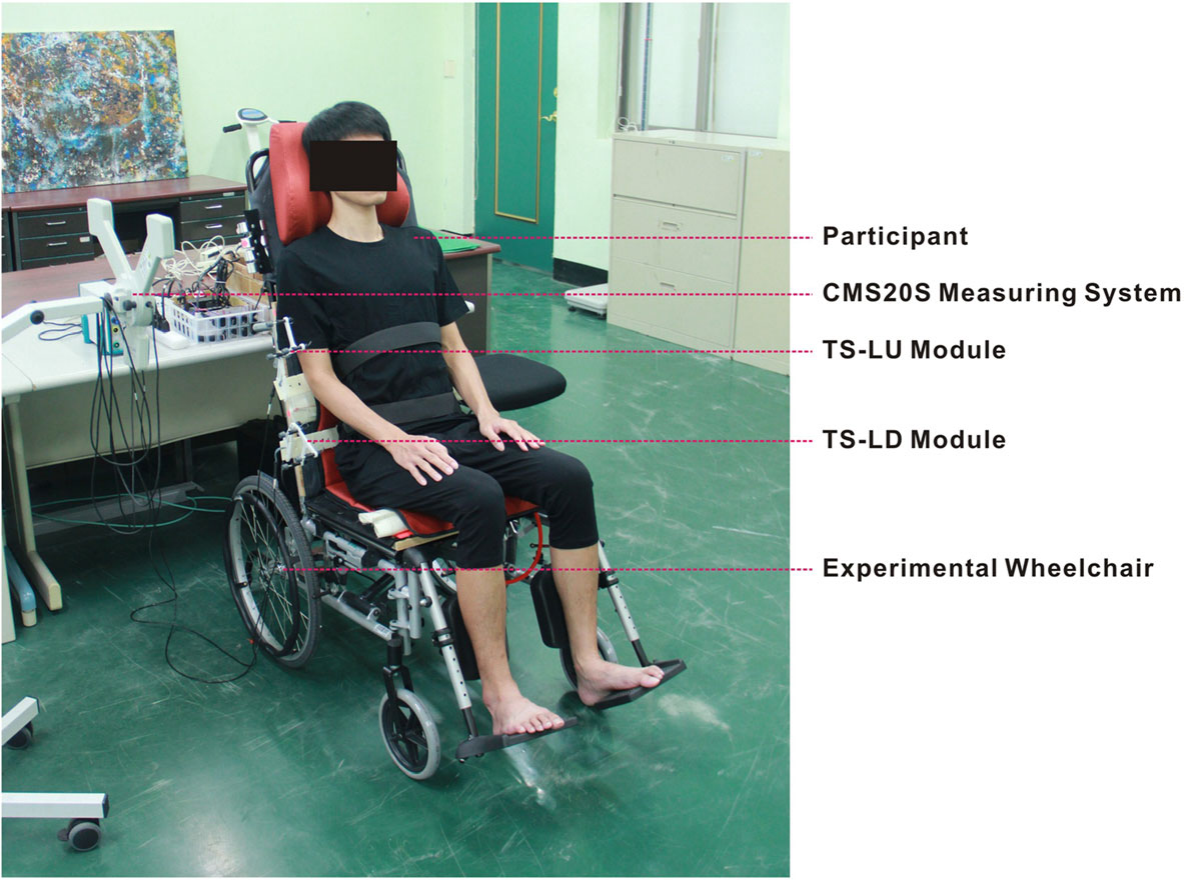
Experimental setup. The picture shows the experimental setup with participant, experimental wheelchair, CMS20S Measuring System, miniature ultrasound transmission modules
